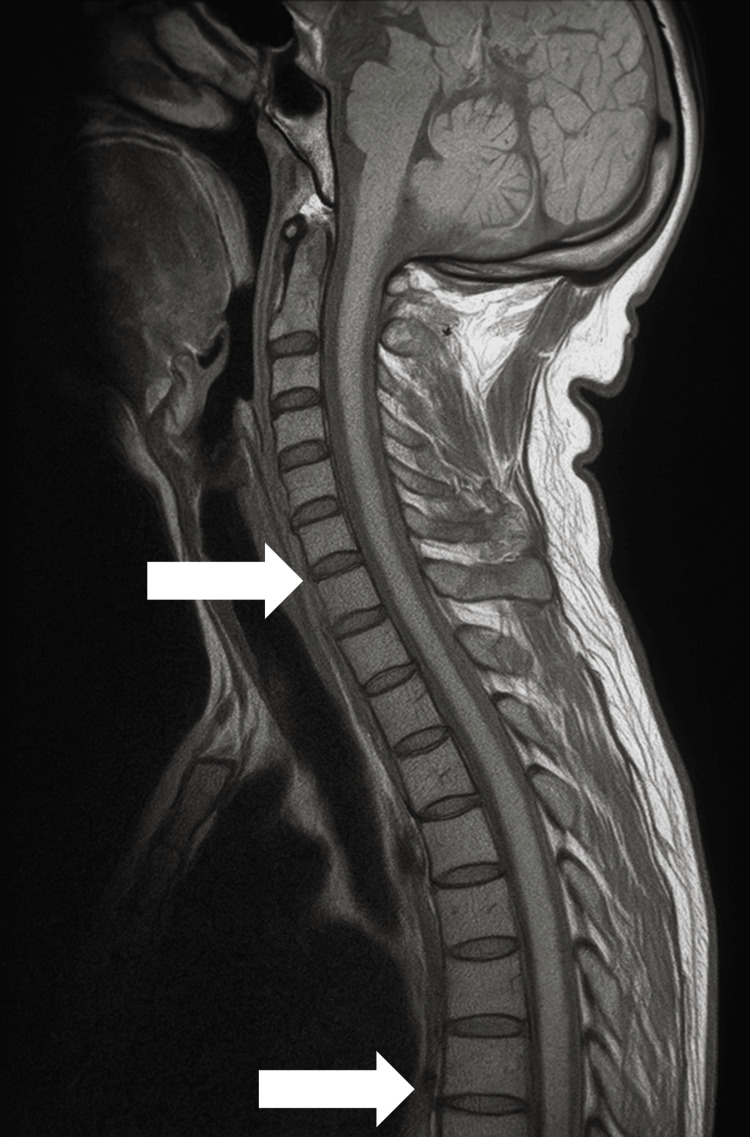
T1-weighted sagittal MRI scan of the cervical spine. Preserved cervical spinal cord with no compressive lesions, intramedullary signal alterations, or significant atrophy. The upper arrow indicates the mid-cervical cord, where spinal morphology and signal are preserved. The lower arrow shows an intervertebral space with no disc protrusion or canal stenosis. No overt signs of cervical myelopathy are visible on this sequence. Thoracic spine imaging was not available for evaluation of spinal cord atrophy often associated with human T-lymphotropic virus type 1-associated myelopathy/tropical spastic paraparesis.
